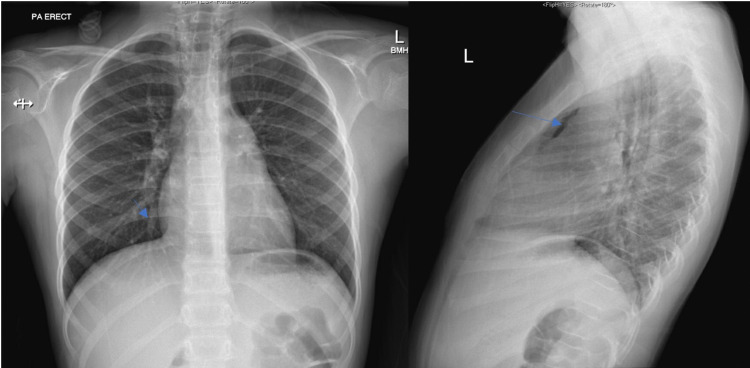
Initial Anterior/Posterior and Lateral Chest X-rays Air tracking into the soft tissues of the neck and continuous air in the superior mediastinum is seen in the anterior/posterior (left) and lateral (right) chest X-rays. No significant pericardial or pleural effusions are noted.
